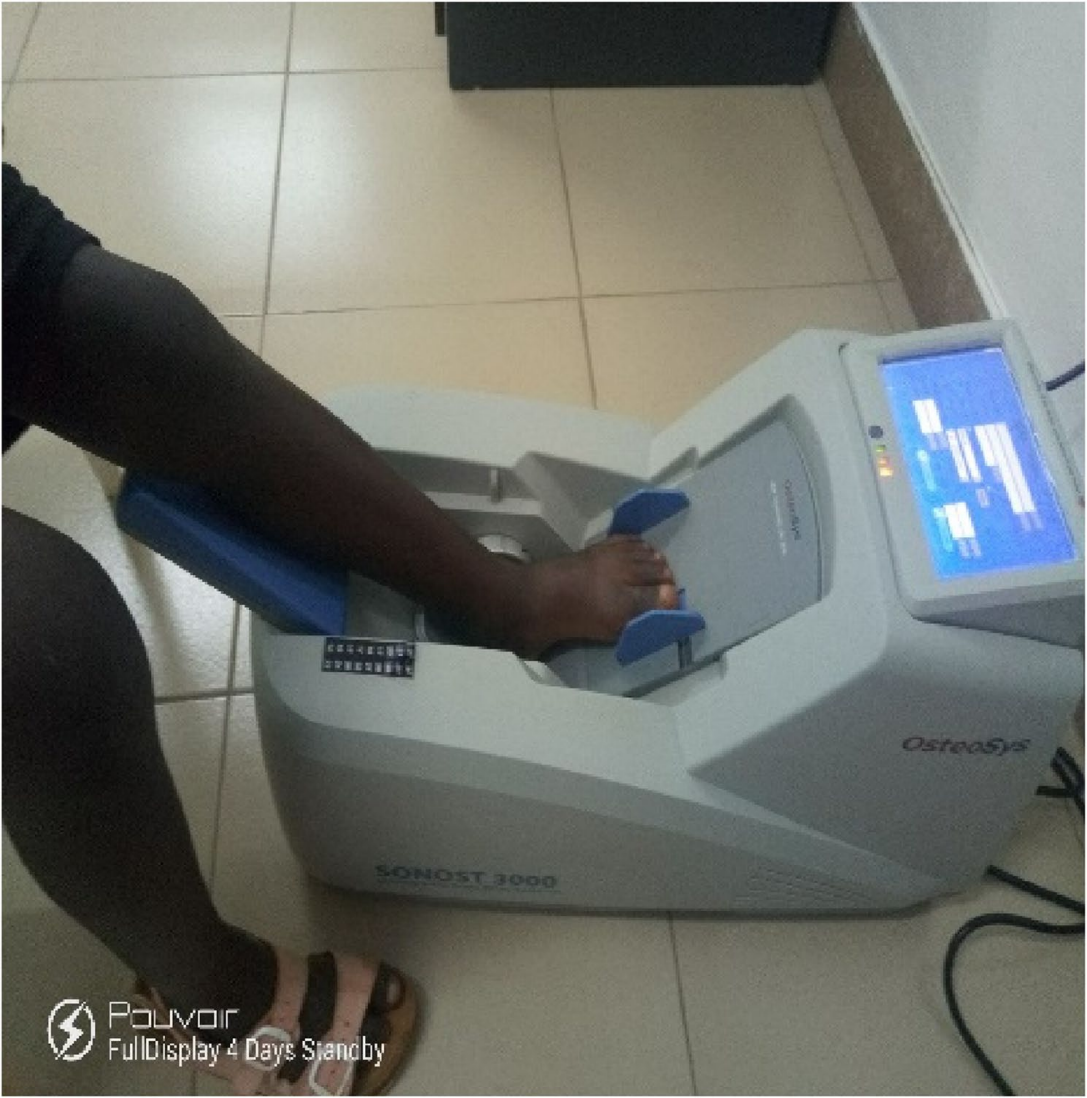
Calcaneal quantitative ultrasound device (*photo taken by the authors*)
